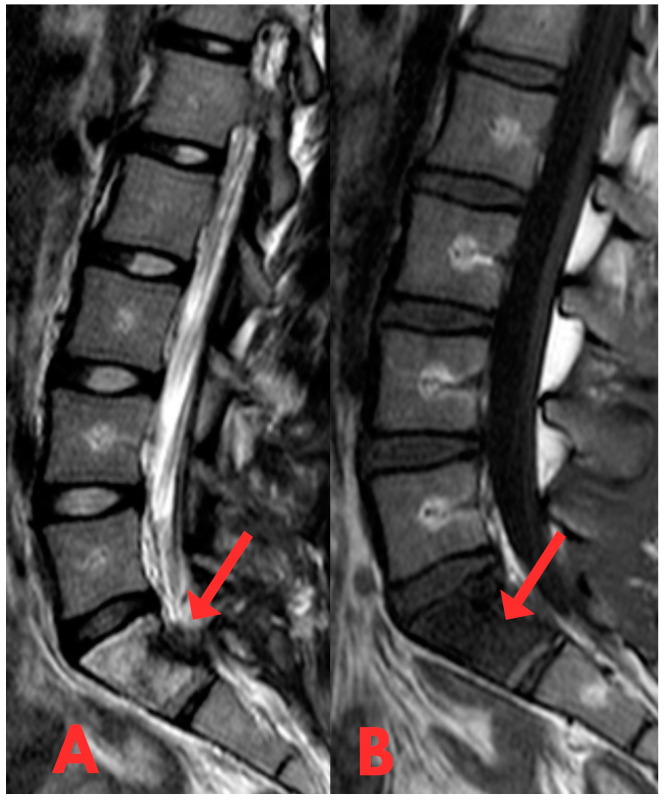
(A,B) Second T1- and T2-weighted sagittal MRI of the lumbar spine (T2W_TSE, T1W_TSE sag) showing no significant change in the S1 lesion. In dynamics, there is a small signal change in the posterior 1/3 of the S1 vertebra body after a biopsy with a small defect.
